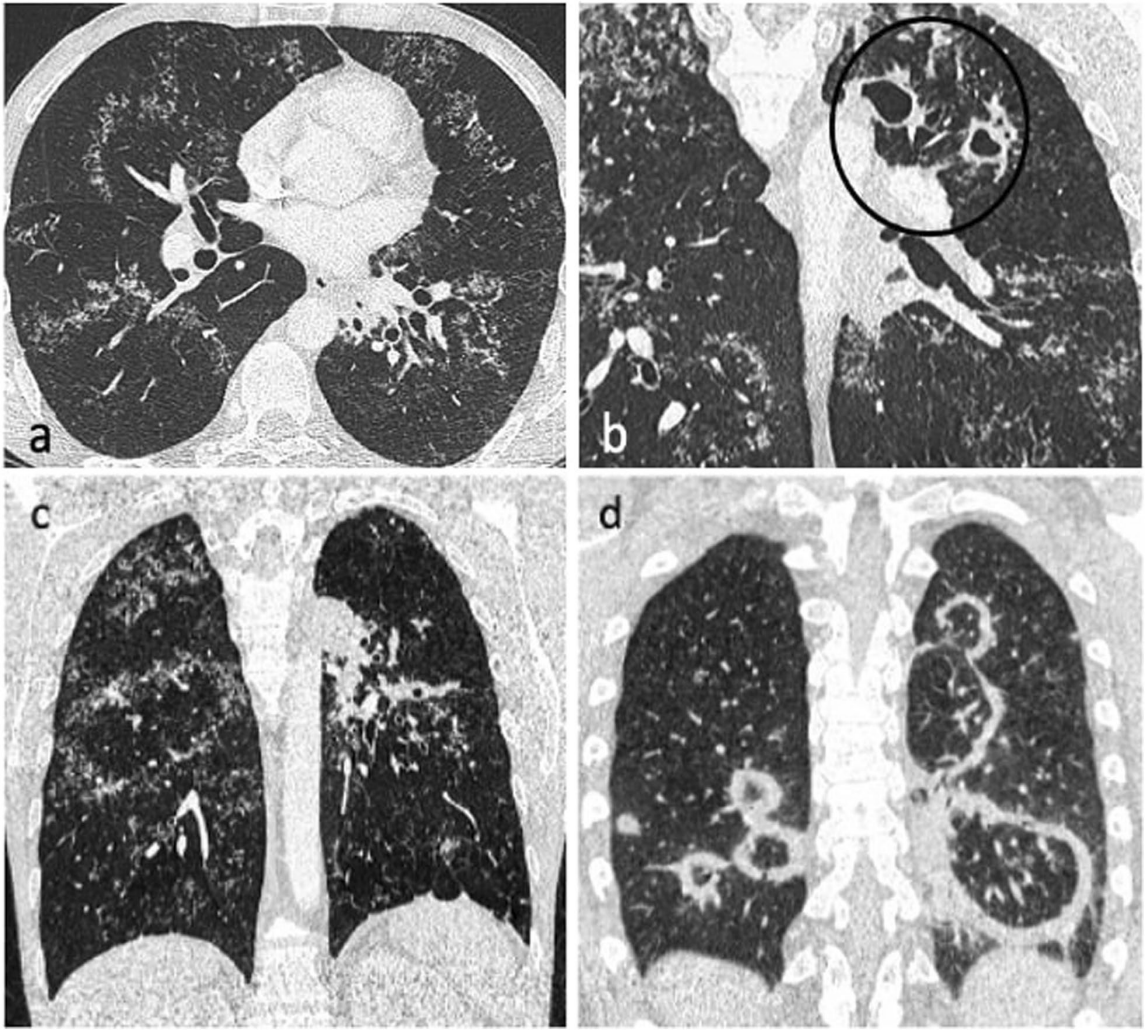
A 37-year-old male with active pulmonary TB presented with a 6-month history of weight loss and night sweats. CT (lung window) demonstrates nodular reversed halo sign in the right lower and middle lobe (axial plane—a, coronal plane—c), and cavitating lesions in the left upper lobe (coronal plane—b, circled). Image (d) is of a 25-year-old male with cryptogenic organising pneumonia who presented with cough and night sweats. CT (lung window, coronal plane) demonstrates multiple areas of rim consolidation reversed halo sign
